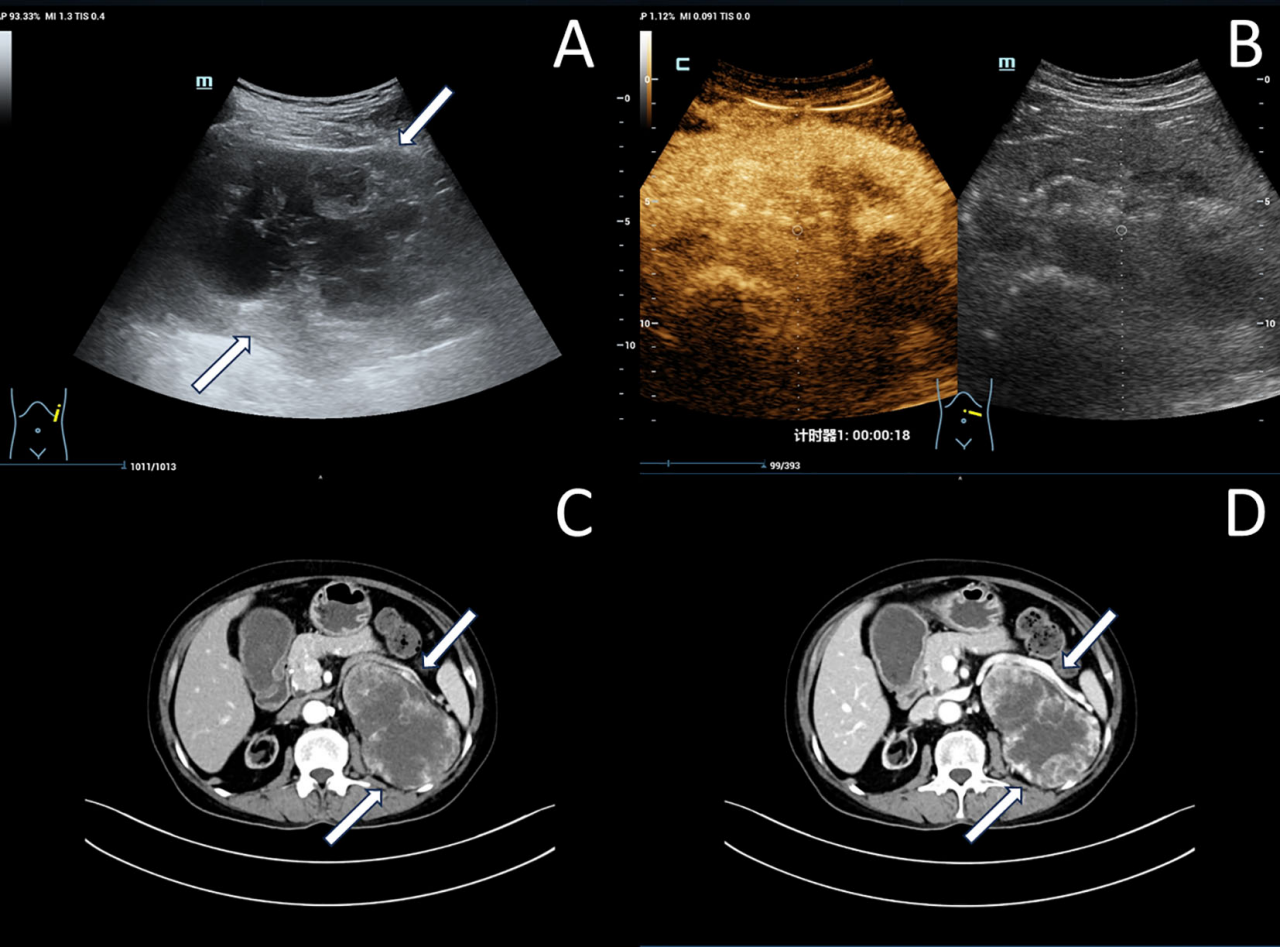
Imaging of left native renal cell carcinoma (Case 1): (A) Grayscale ultrasonography reveals a large cystic-solid mass in the left native renal region (white arrow). (B) Contrast-enhanced ultrasonography demonstrates heterogeneous hyperenhancement within the mass. (C, D) Enhanced computed tomography scan shows heterogeneous hyperenhancement within the mass (white arrow).
